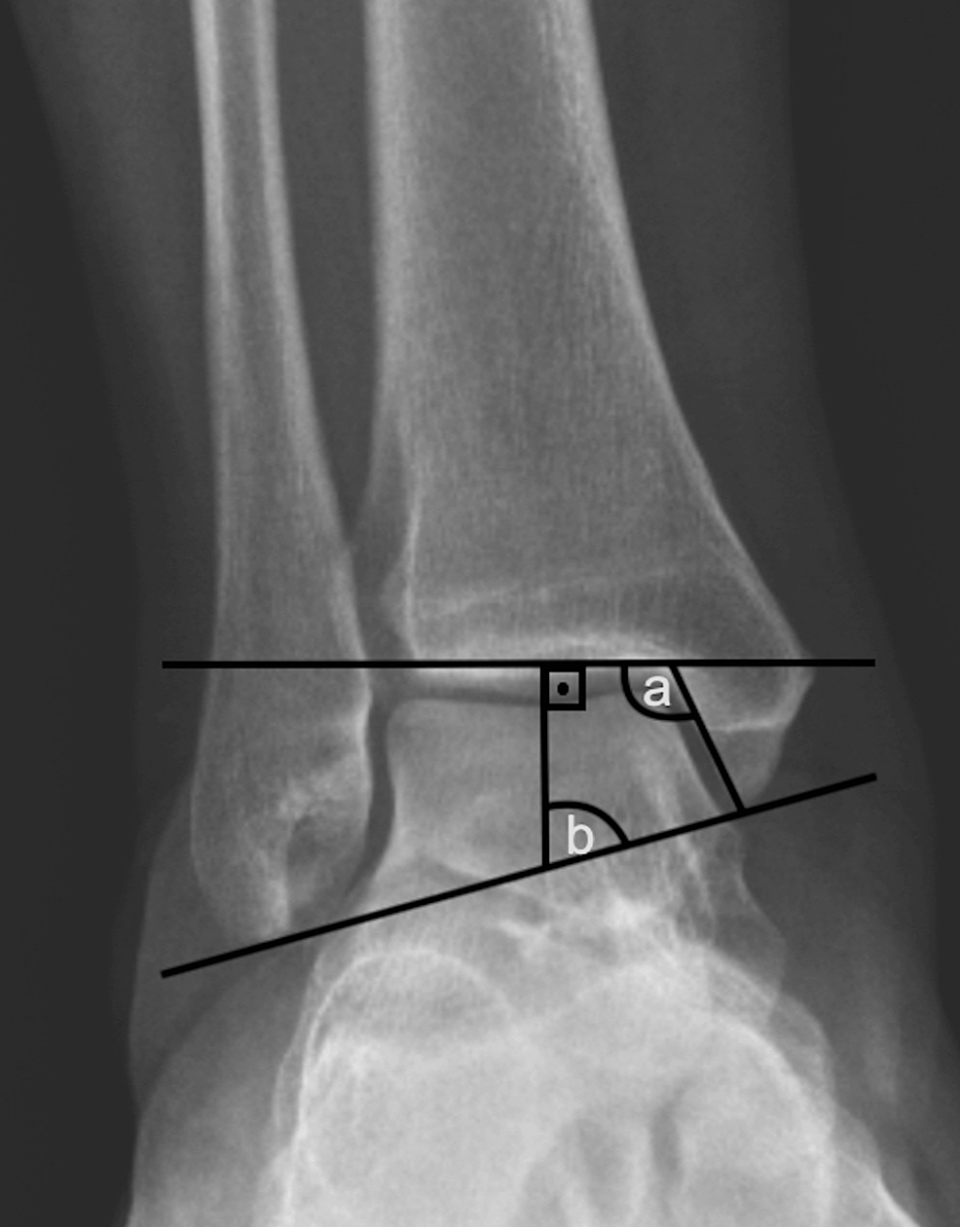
Measurements evaluated on anteroposterior ankle radiographs. a. Medial malleolar slip angle, b. Talocrural angle.
